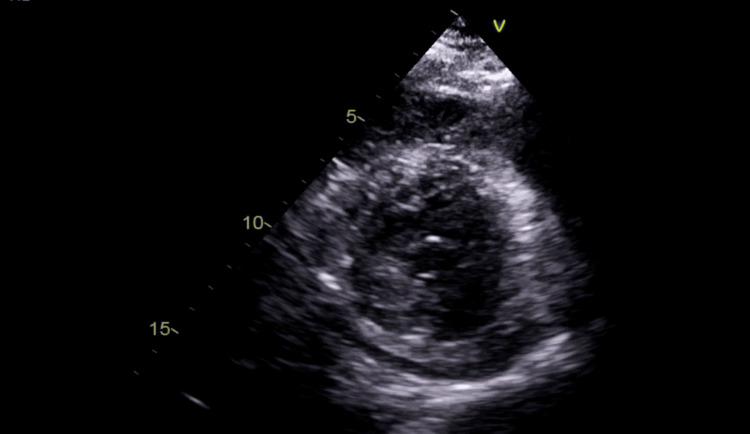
Echocardiography demonstrating pericardial effusion Initial transthoracic echocardiogram demonstrated global hypokinesia and pericardial effusion.
